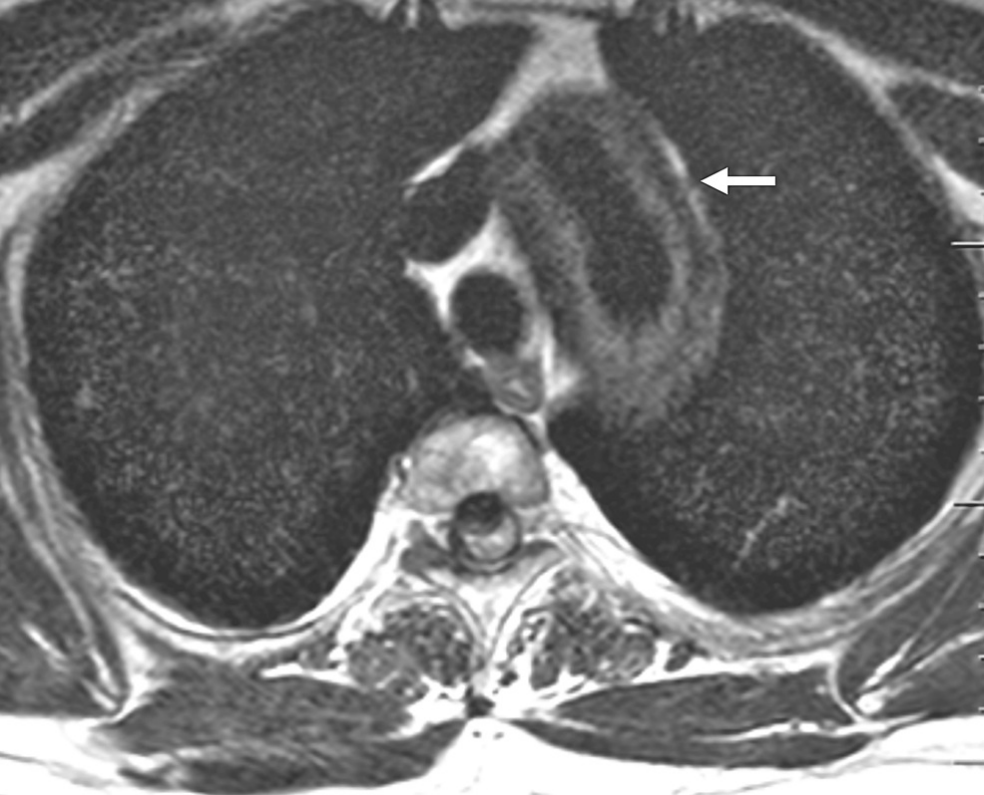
Preoperative MRI (T2 axial) showing a dilated thoracic aorta with a periaortic soft tissue mass (arrow).
